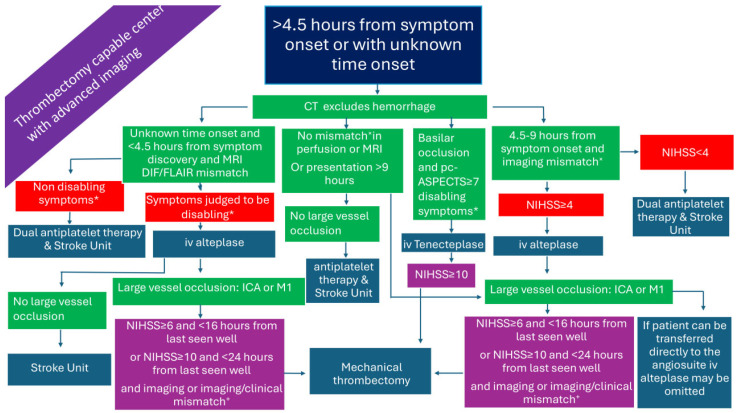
Management of AIS patients presenting >4.5 h from symptom onset in a thrombectomy-capable center with advanced imaging. AIS: acute ischemic stroke; ICA: internal carotid artery; iv: intravenous; M1: M1 segment of the middle cerebral artery; M2: M2 segment of the middle cerebral artery; NIHSS: National Institutes of Health Stroke Scale; pc-ASPECTS: posterior circulation Alberta stroke program early CT score. * symptoms that interfere with daily living and previous activities of the patient. ^×^ Perfusion lesion–ischemic core mismatch ratio > 1.2, absolute difference in volume > 10 mL, and ischemic-core volume < 70 mL. ^+^ penumbral volume to infarct volume of ≥1.8, with an absolute penumbral volume of ≥15 mL and an estimated volume of the ischemic core < 70 mL (DEFUSE-3 [54]), or ≥80 years old and NIHSS ≥ 10 and infarct core < 21 mL, or <80 years old and NIHSS ≥ 10 and infarct core < 31 mL, or <80 years old and NIHSS ≥ 20 and infarct core 31 = 51 mL (DAWN [55]).
